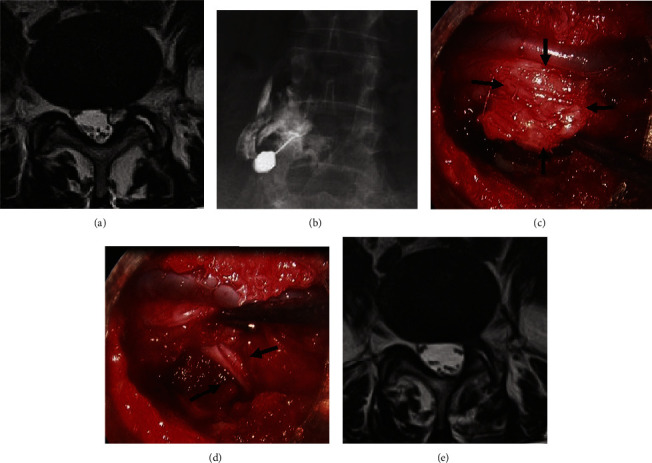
The case with failed rupture of the cyst: (a) pretreatment MRI, (b) failed rupture of the cyst, (c) before cyst resection (arrow; cyst), (d) after cyst resection (arrow: L5 root), (e) postoperative MRI. MRI, magnetic resonance imaging.
